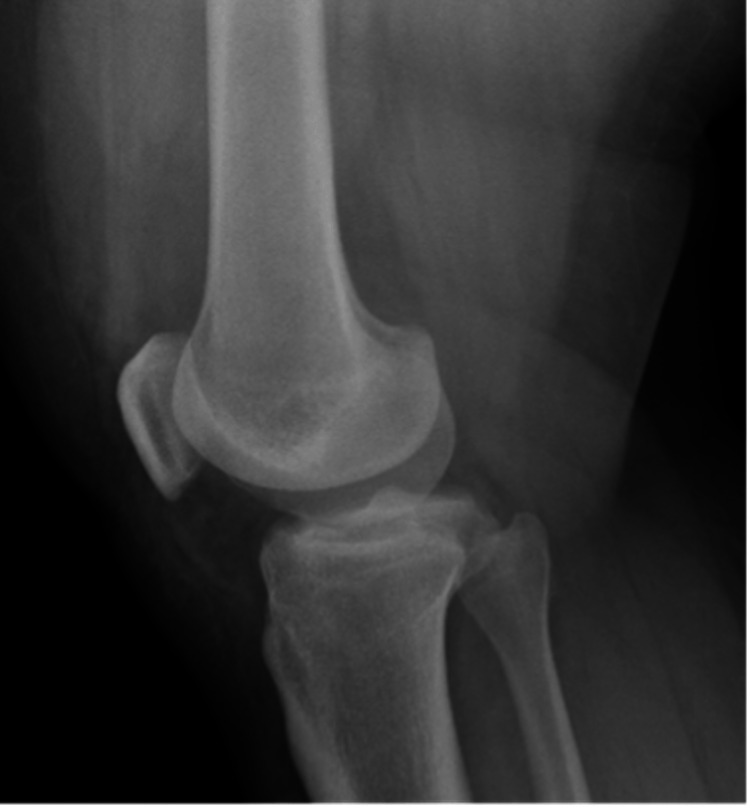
Lateral radiograph of the left knee showing a patellar dislocation
